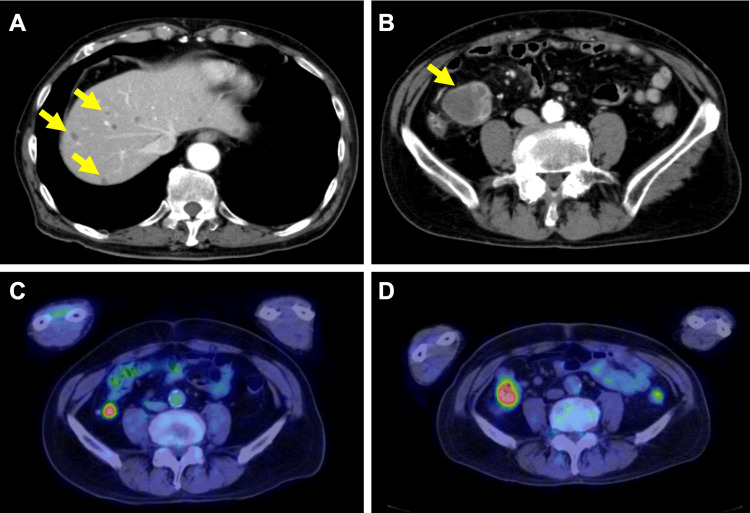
Imaging findings after maintenance therapy and comparison with pre-treatment images FDG-PET: fludeoxyglucose-18-positron emission tomography (A,B) Contrast-enhanced CT after two cycles of maintenance therapy: Multiple low-density areas in the liver (suspected metastases, yellow arrows). Dilatation, wall thickening, and deformation of the terminal ileum are visible. (C) FDG-PET before treatment initiation. (D) FDG-PET after two cycles of maintenance therapy. The area of abnormal FDG uptake in the terminal ileum has increased in size, indicating disease progression at this site
